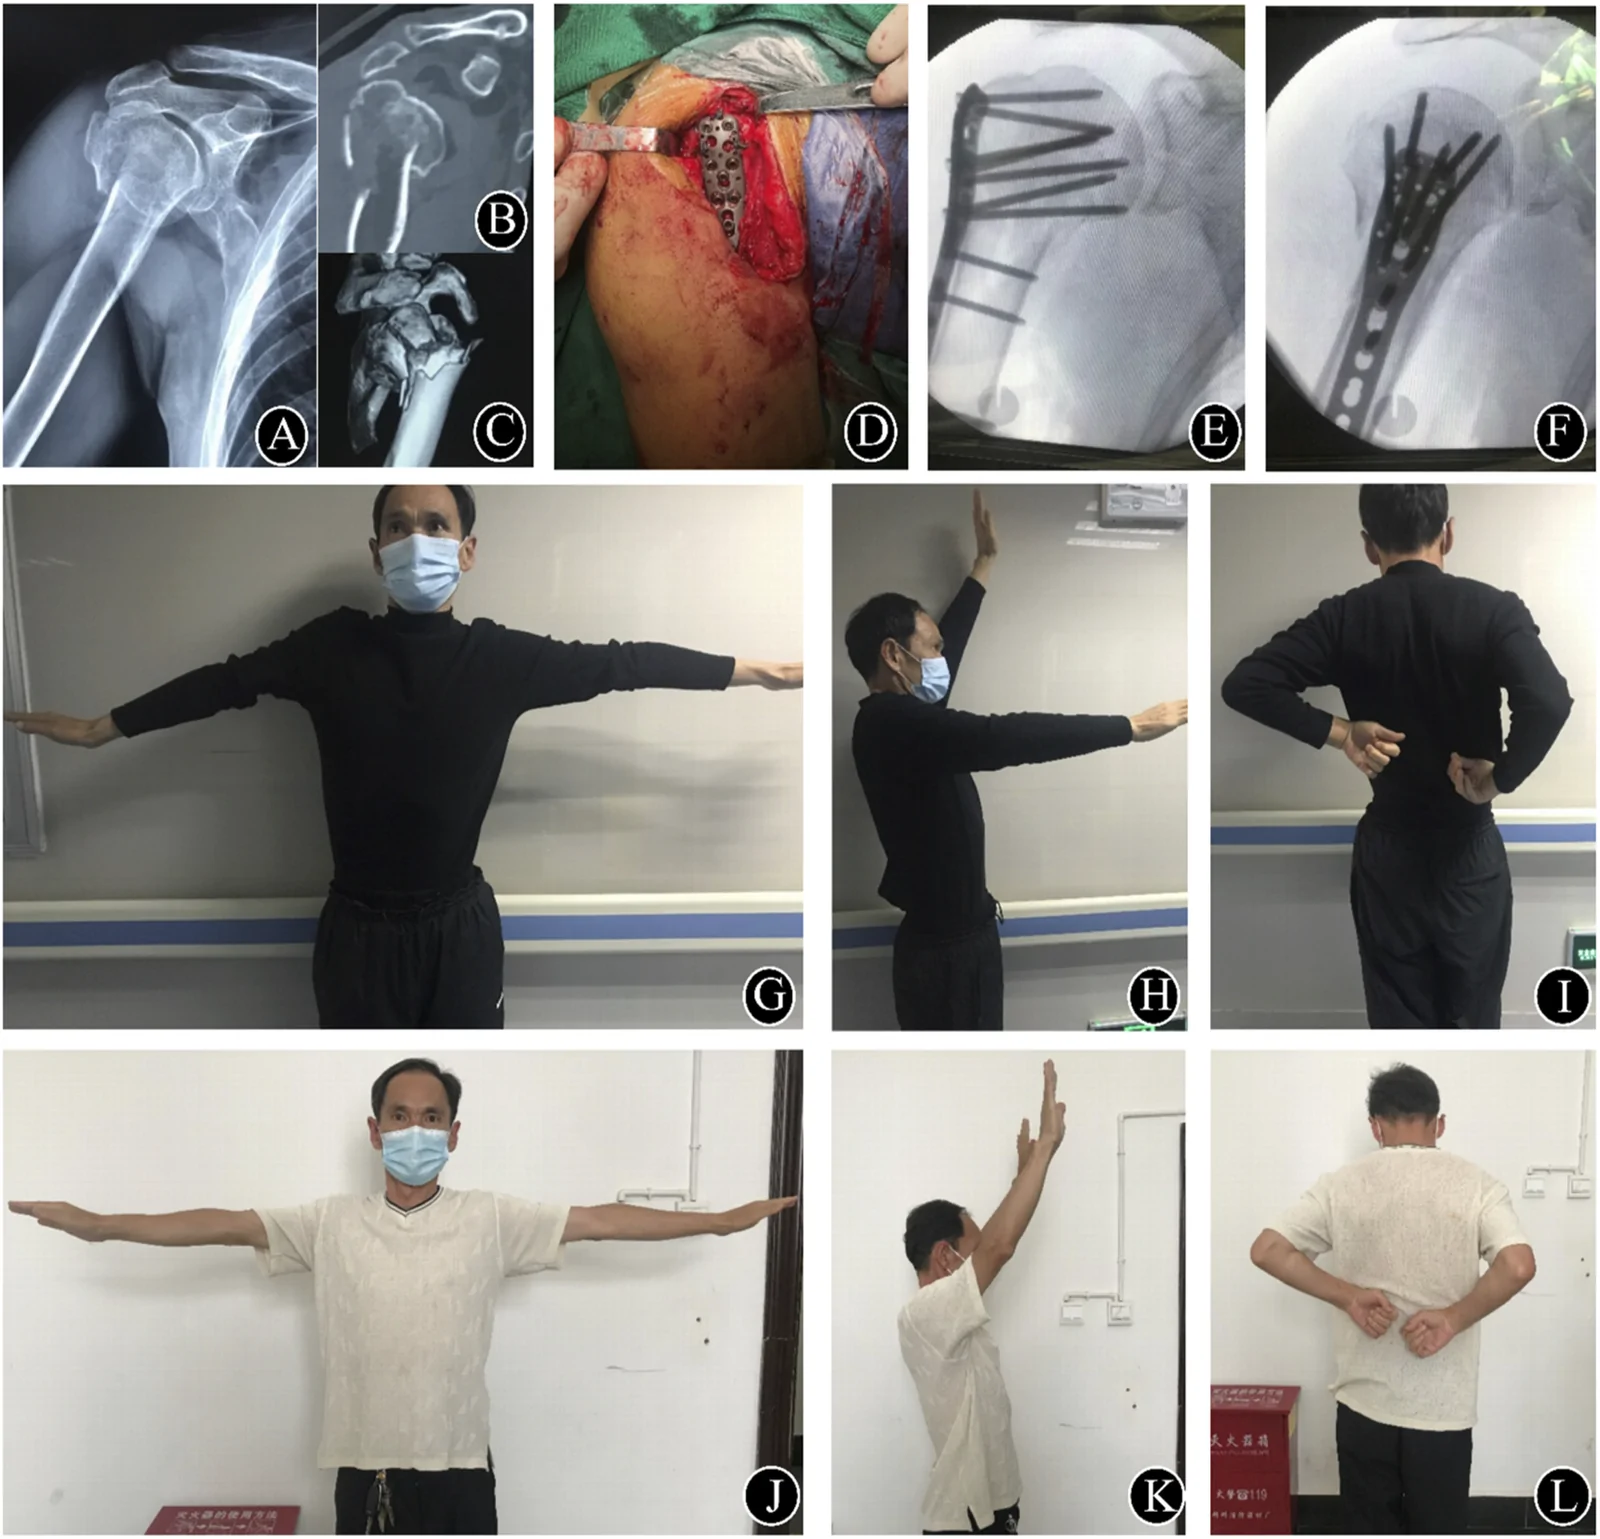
Case 2: PHILOS plate (50-year-old man, Neer four-part fracture). (A–C) preoperative radiographs and CT; (D–F) intraoperative fluoroscopy; (G–I) 3-month — restricted motion; (J–L) 6-month — substantial functional recovery.
